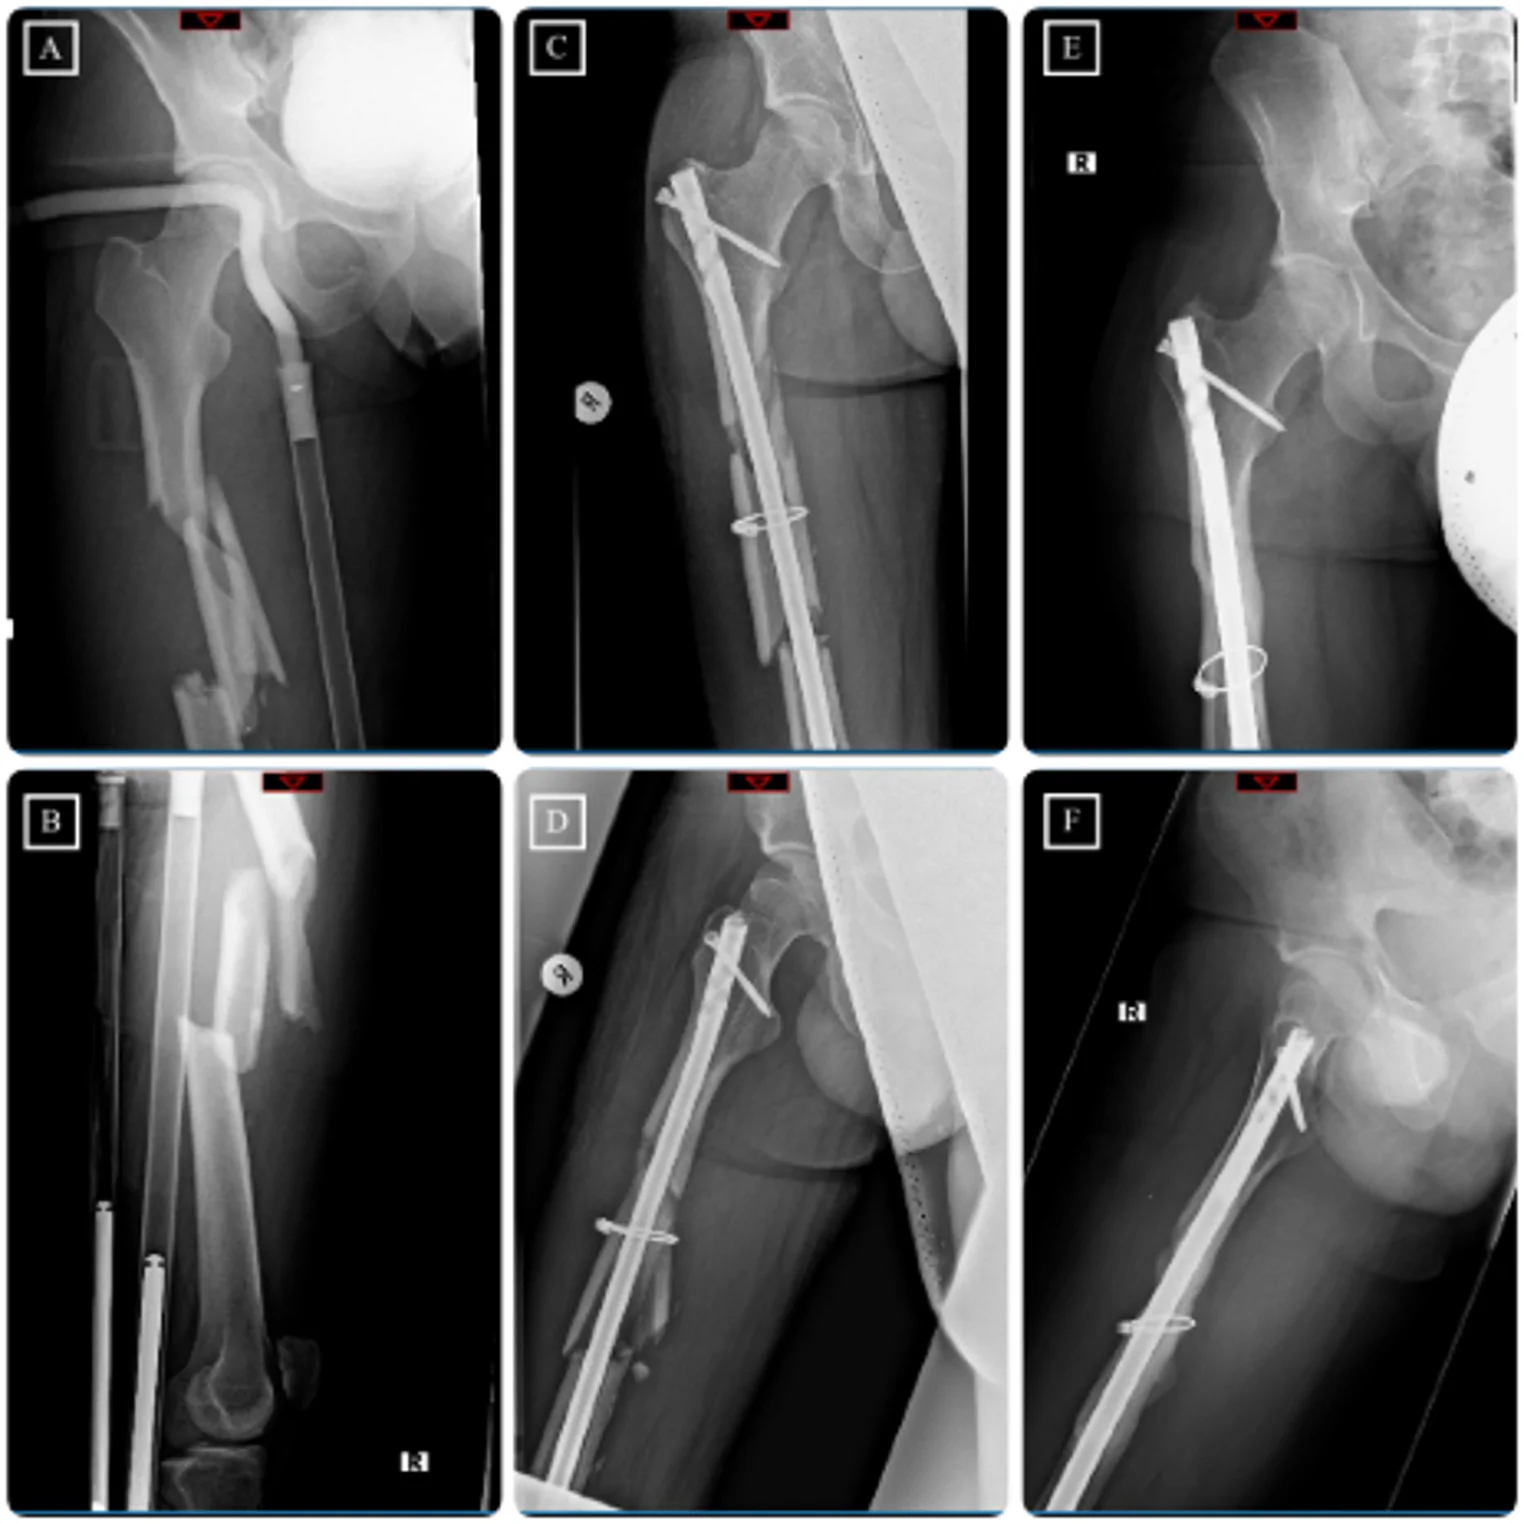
Radiographic Sequence of Midshaft Femur Fracture Treated Primarily by Residents, Complicated by Nonunion and Revised Successfully. A–B: Initial injury radiographs showing a closed midshaft femoral fracture.C–D: Postoperative images following open reduction and internal fixation with intramedullary nailing and cerclage wiring, performed autonomously by orthopedic residents.E–F: Follow-up radiographs one year later, after revision surgery for nonunion, showing radiographic signs of healing and fracture union
